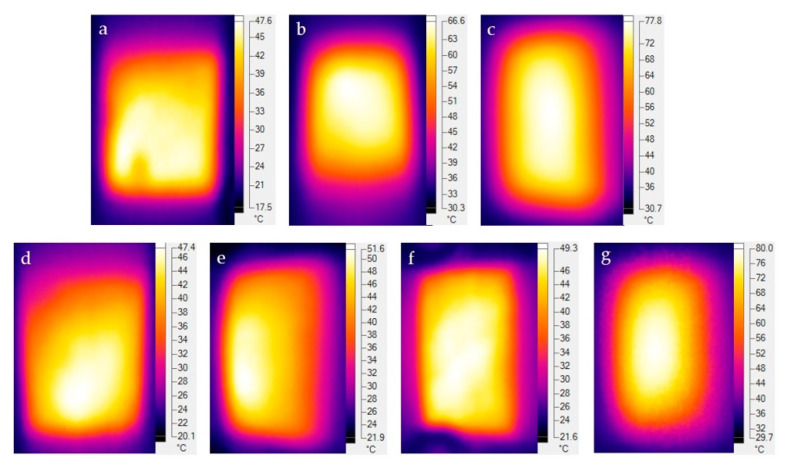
Infrared thermogram of CCGA prepared via various ultrasonic conditions of (a) 600 W, 40 min; (b) 800 W, 40 min; (c) 1000 W, 40 min; (d) 1000 W, 10 min; (e) 1000 W, 30 min; (f) 1000 W, 50 min; (g) 1000 W, 70 min.
